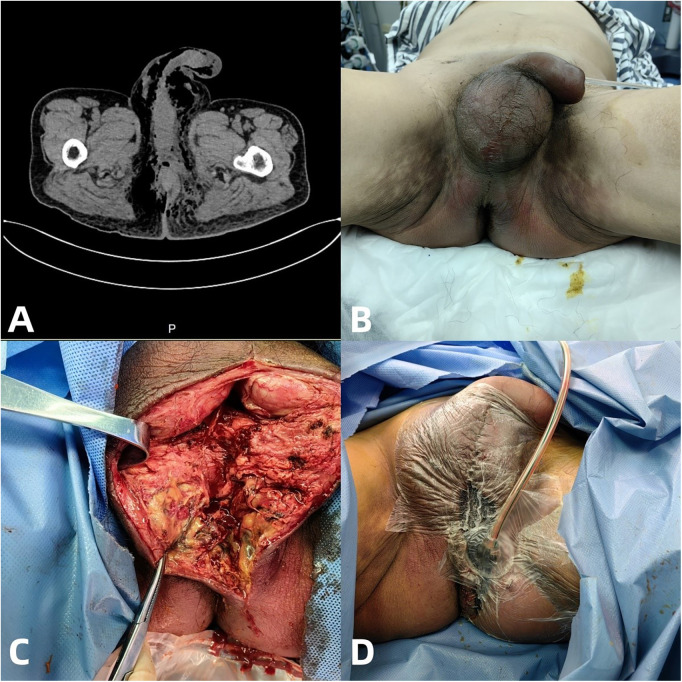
(A) Emergency enhanced CT scan of the entire abdomen indicates a discontinuity in the left wall of the rectum, with surrounding exudate, fluid accumulation, thickening of the fascia around the rectum, exudate, and gas accumulation around the pelvic floor and perineum, and multiple gas accumulations in the groin area, pelvic wall, and left abdominal cavity on both sides. (B) Physical examination revealed extensive redness and swelling around the scrotum and anus, extending all the way to both buttocks and at the base of the thighs. The scrotum and penis were also highly swollen. (C) During the emergency perineal incision and drainage surgery, the scrotum and perineal anal soft tissue were fully drained, and a large amount of feces and pus were extracted. (D) Vacuum assisted closure (VAC) after perineal incision and drainage surgery.
